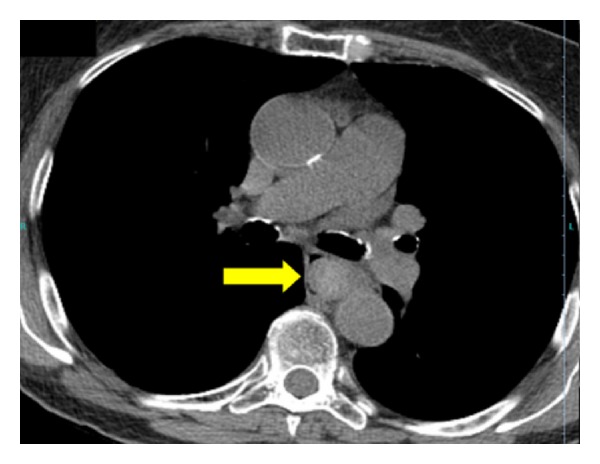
CT of middle esophagus revealing a large intraluminal hematoma obstructing the lumen. Notice that there is only a thin layer of air between the hematoma and esophageal wall (arrow).
